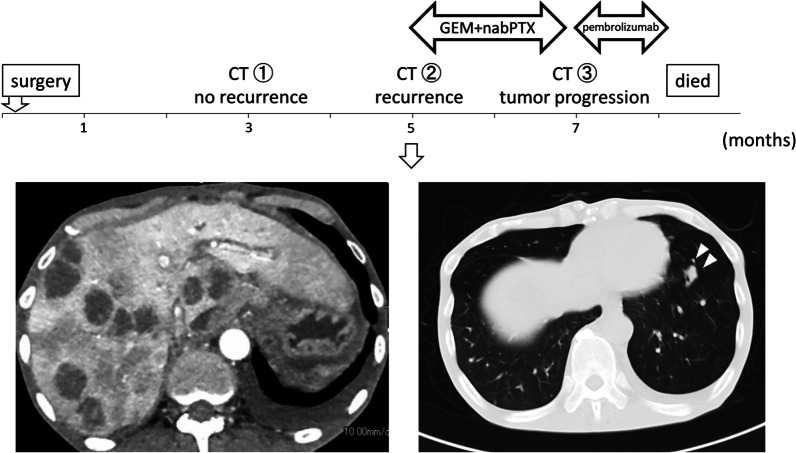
Summary of the treatments. Five months after surgery, a CT scan revealed the presence of multiple liver, lung, and lymph node metastases and peritoneal dissemination. Chemotherapy with gemcitabine (GEM) plus nab-paclitaxel (nabPTX) was administered. Subsequently, pembrolizumab was administered as the second-line treatment, however, did not show a good response
